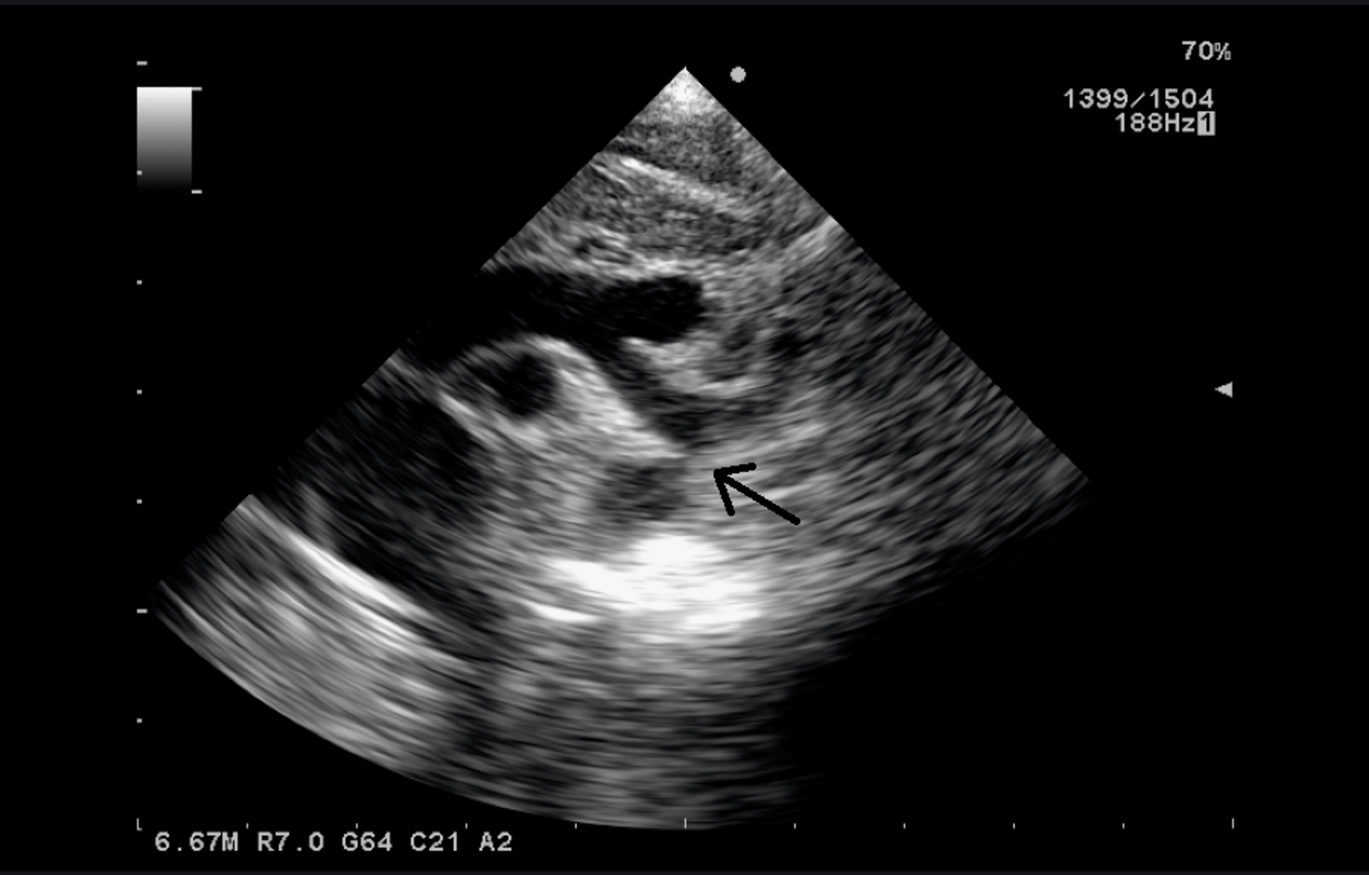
Echocardiography (Eco 2D/ Doppler) on day 1 of life (Case 1): a “gothic” aortic arch with isthmus narrowing (arrow).
